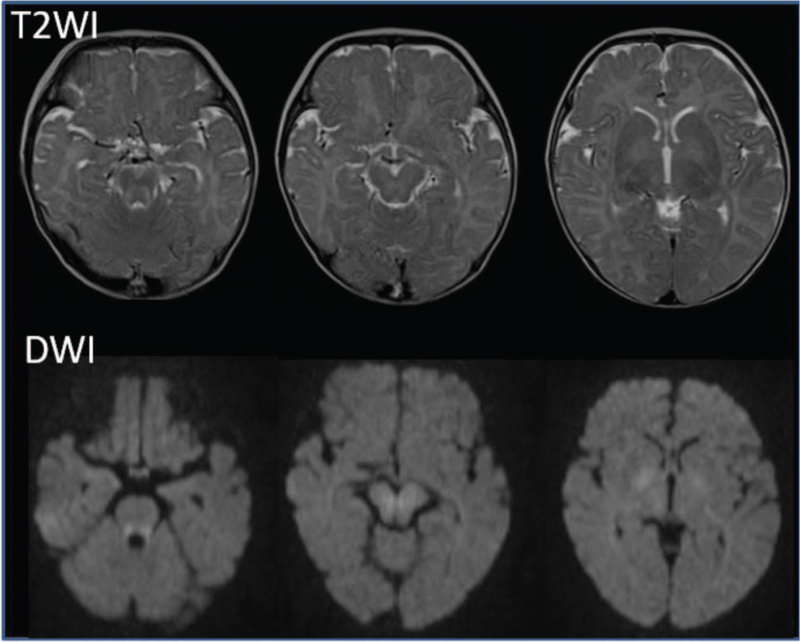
Initial MRI on the third hospital day shows multifocal, symmetric lesions within the superior cerebellar peduncle, lateral pons, thalami, posterior limb of internal capsule on DWI and T2-weighted image. DWI = diffusion-weighted imaging, MRI = magnetic resonance imaging.
